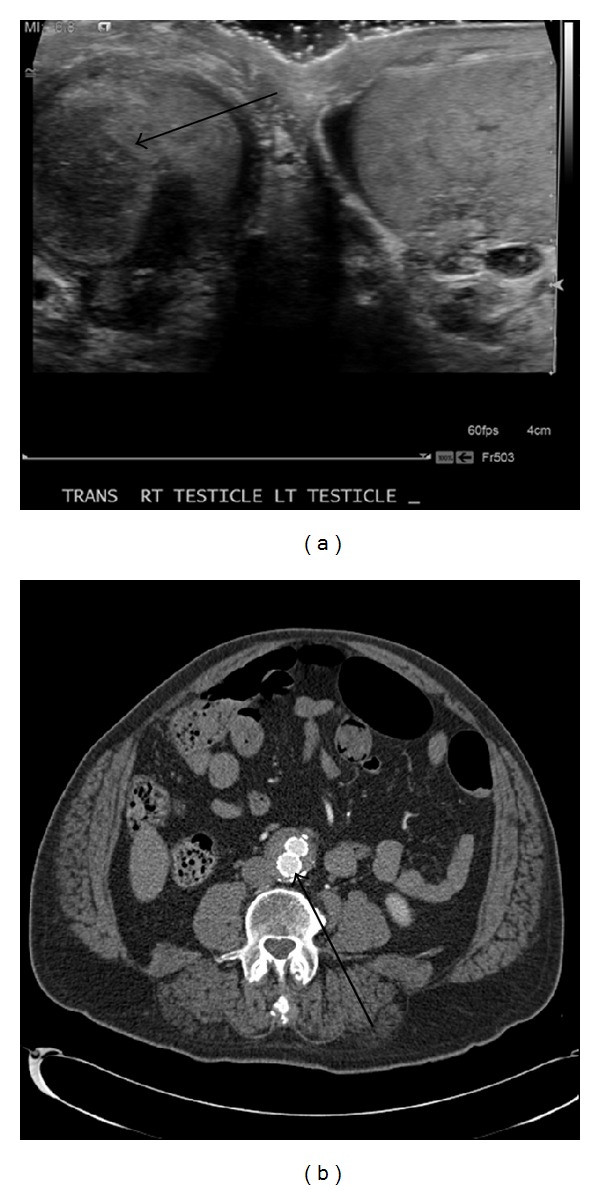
(a) Testicular ultrasound demonstrating decreased echogenicity within the right testicle (arrow). (b) Computed topographic angiogram (CTA) showing endovascular stent graft (arrow).
